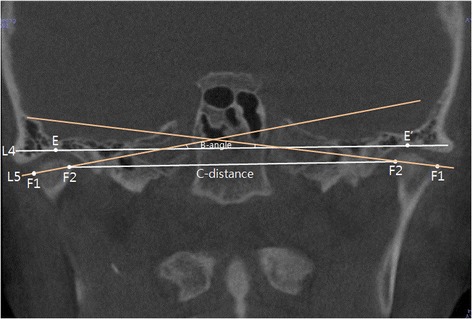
Reference points and measurement of coronal view in CBCT. E,E′ highest point with joint, F1 outer pole of condylar head, F2 inner pole of condylar head, L4 line passing E and E′, L5 line passing F1 and F2, B-angle angle between L4 and L5, C-distance distance between left F2 and right F2
